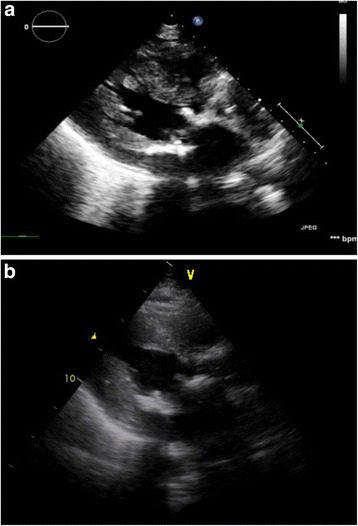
2-D transthoracic echocardiography. a Pre-septal ablation. b Post-septal ablation showing septal scar and remodeling with relief of LVOT obstruction
